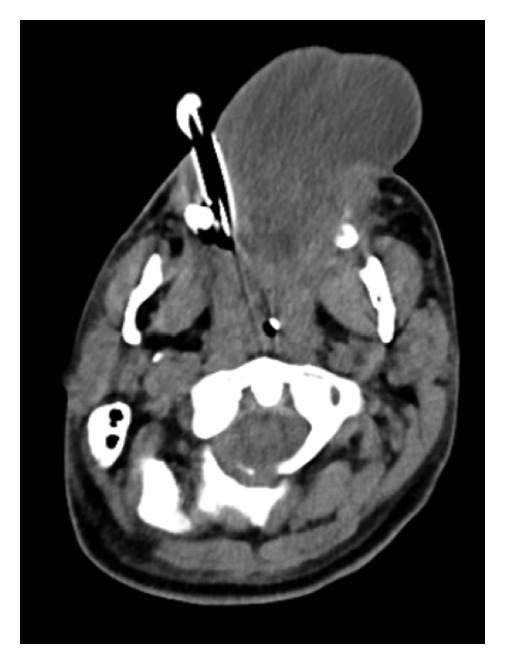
Axial cross section of a soft-tissue window computed tomography image with a tongue protrusion and endotracheal tube in place.
